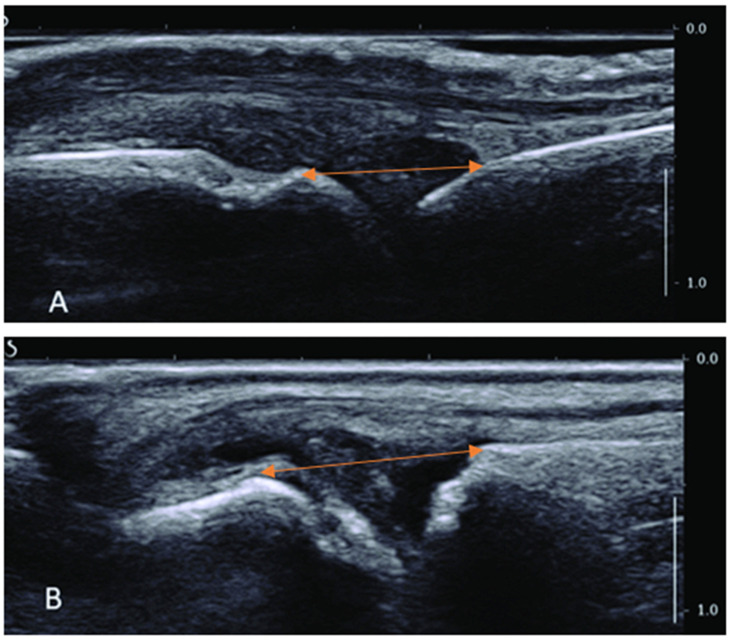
Musculoskeletal ultrasound images. Image (A) is dorsal side of MCP II, and Image (B) is dorsal side of MTP II. Orange line indicates measured joint distance.
